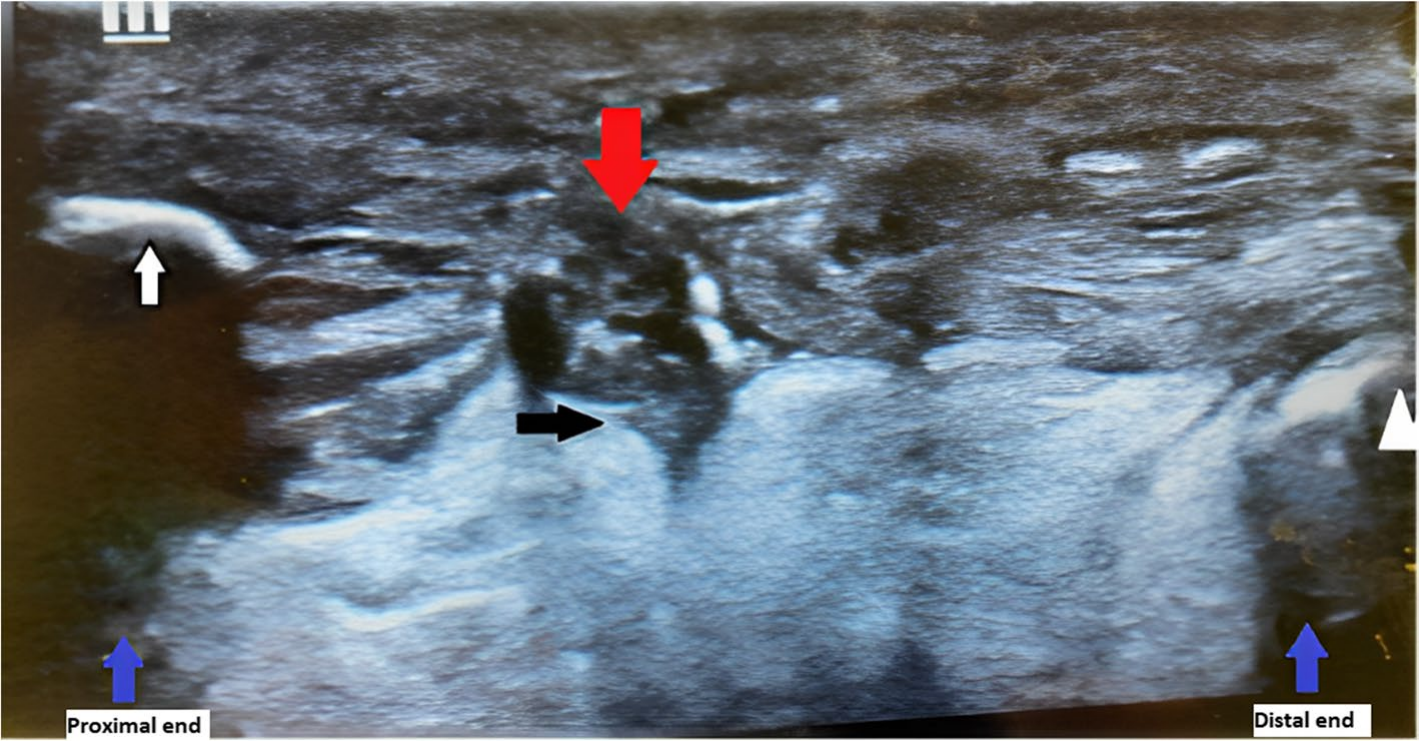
Point-of-care ultrasound with a longitudinal view of the Achilles tendon. The white arrow indicates an avulsed fragment of calcaneum, the black arrow indicates the torn edge of the Achilles tendon, and the white triangle indicates calcaneum. Blue arrows indicate the proximal end and distal end (left to right). The red arrow indicates a possible hematoma between the torn edges of the Achilles tendon
